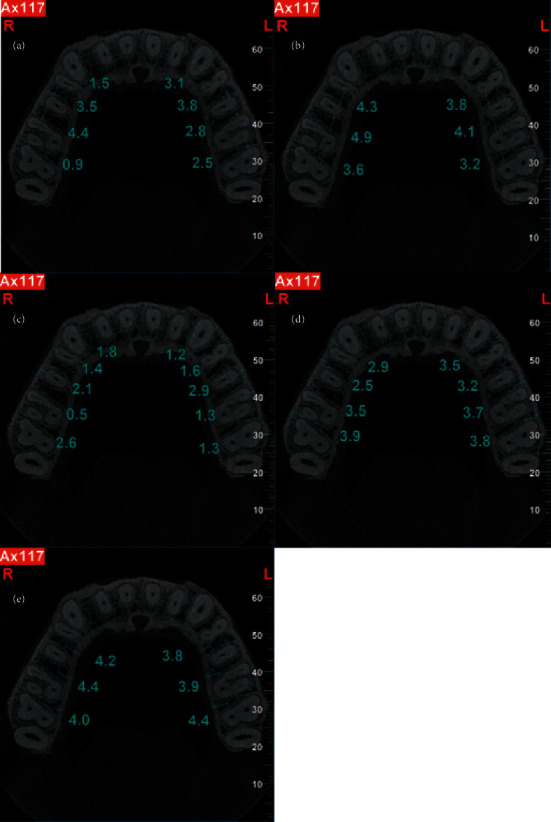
Axial CBCT scans demonstrating measurement (in millimeter) of interradicular distance, cortex thickness, and safe depth of miniscrew insertion. (a) Distance between buccal roots. (b) Distance between palatal roots. (c) Buccal cortex thickness. (d) Safe buccal depth. (e) Safe palatal depth.
